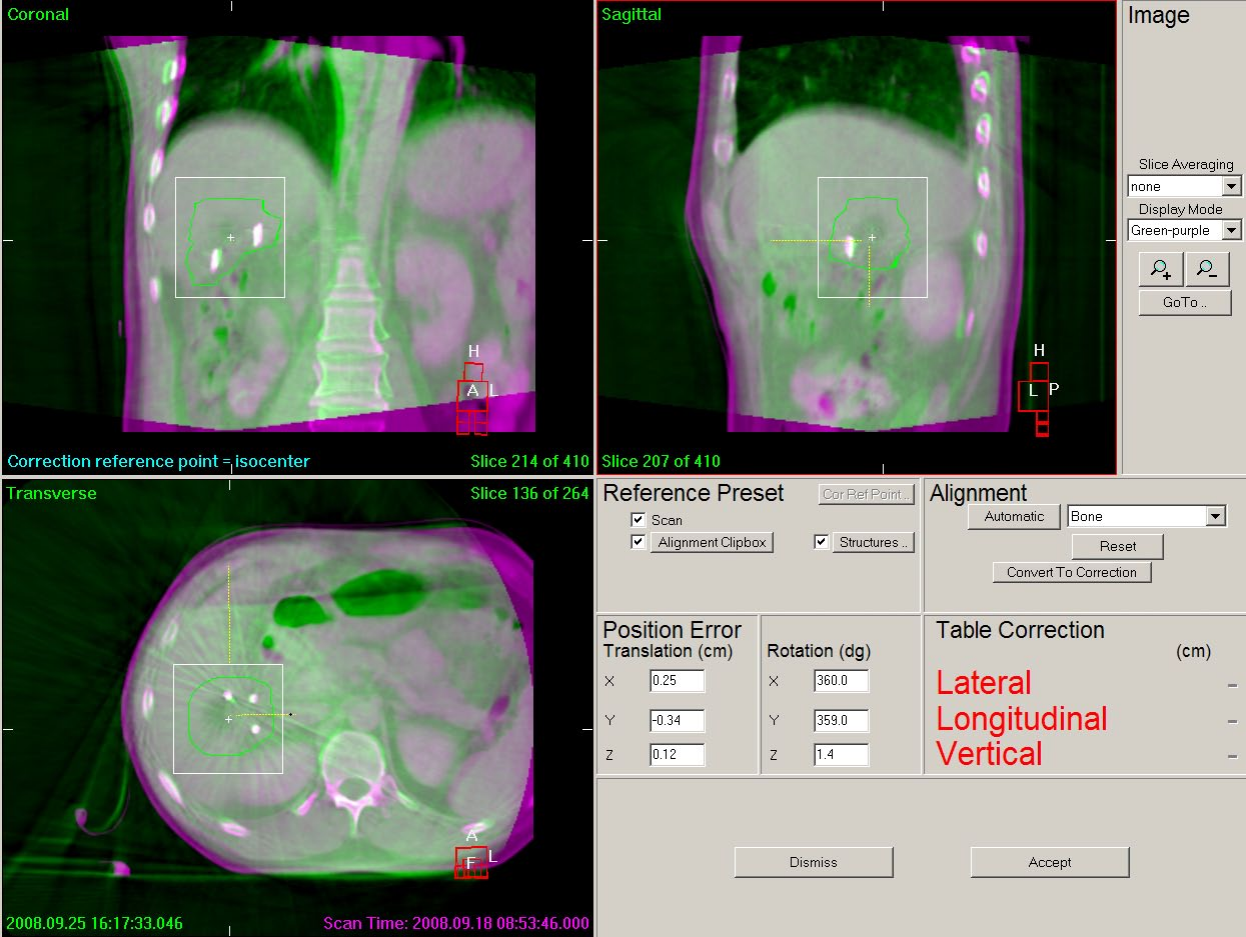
Fiducial marker match in which CBCT images are shown in green and reference CT images are shown in purple. The white line is clipbox, which included the fiducial markers in the tumor bed and excluded the ribs and vertebral bodies. Fiducial markers were white when the markers of two image sets were completely fit.
